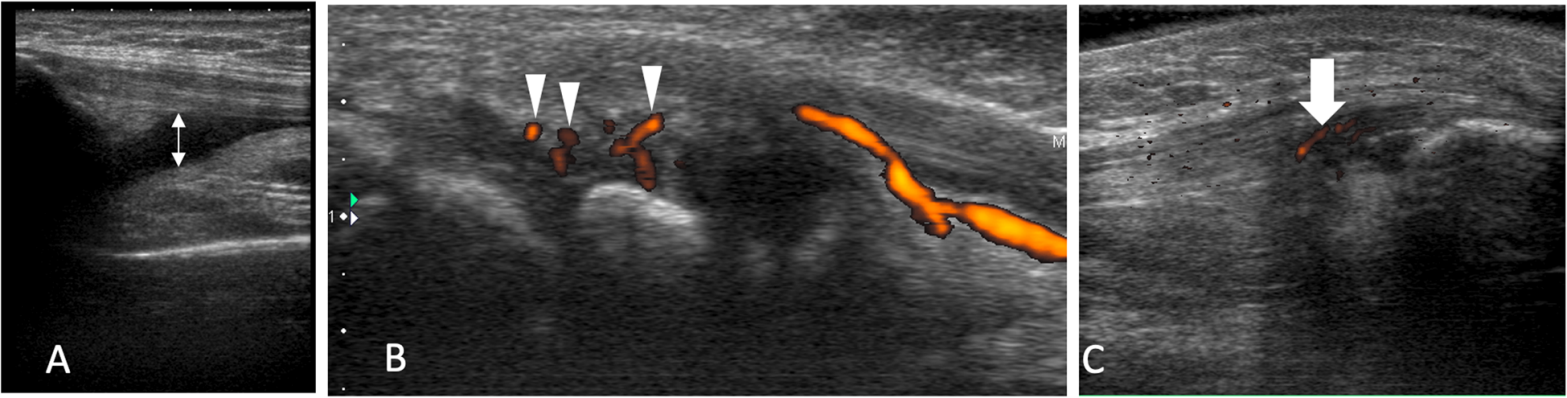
MSUS features in JIA. a Effusion (double-headed arrow) in the suprapatellar pouch of the knee. b Synovial hypertrophy with PD signals (arrowheads) in the radiocarpal and intercarpal joint. c Longitudinal ultrasound image of the patellar tendon that shows hypoechogenicity and PD signals (arrow) inside the enthesis
